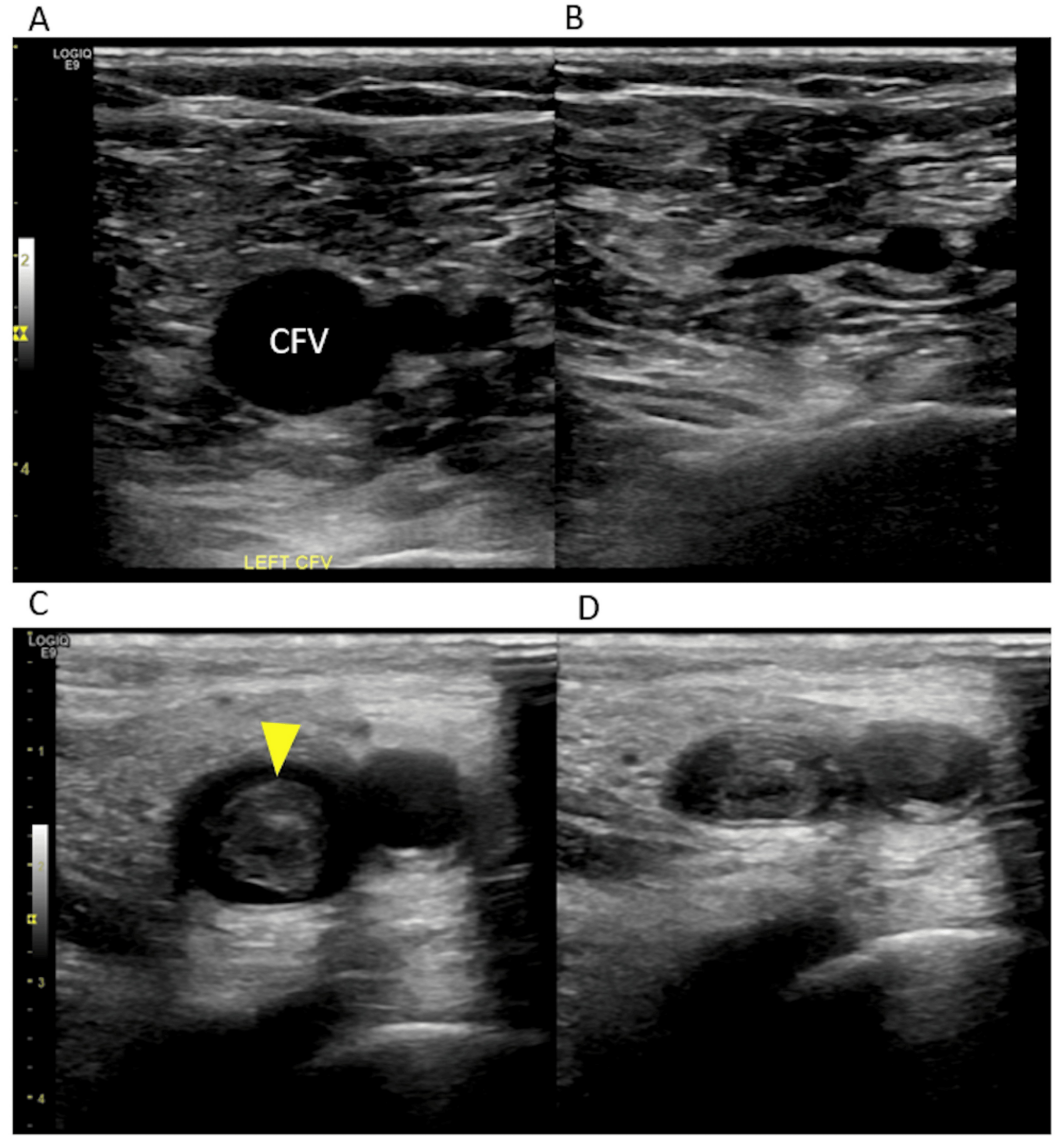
Compression ultrasound for evaluating lower extremity DVT (A) Normal common femoral vein that is fully compressible with transducer pressure (B). (C) Common femoral vein with a hyperechoic thrombus in the lumen (arrow), which is non-compressible (D). DVT: deep vein thrombosis Image credit: Abhilash Koratala
